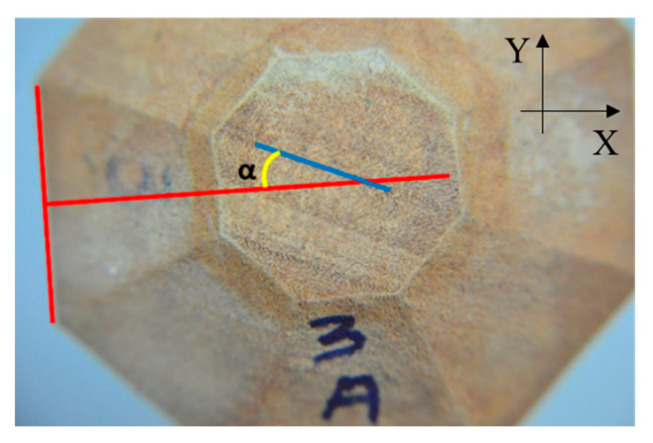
Direction of the growth ring (blue line) and radial (R) and tangential (T) directions parallel to the Y and X axes (red lines), with the red line crossing the cross section indicative of the direction of propagation of the ultrasound wave.
